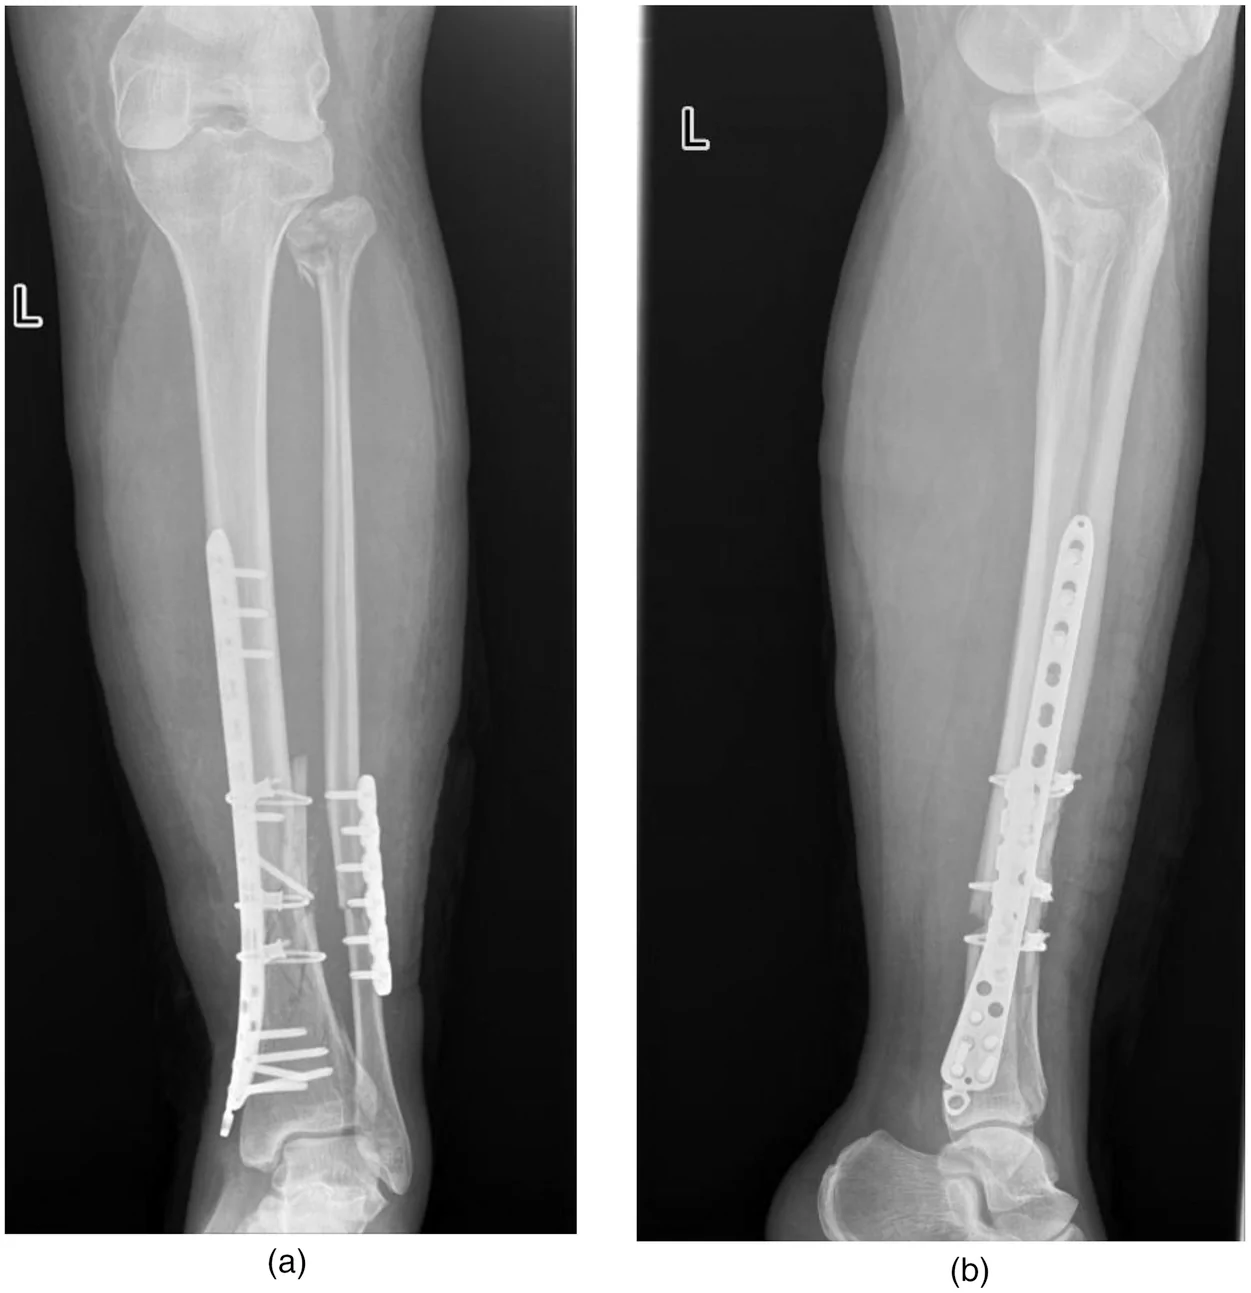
(a,b) on postoperative follow-up x-ray, satisfactory alignment of the fracture fragments is observed.
